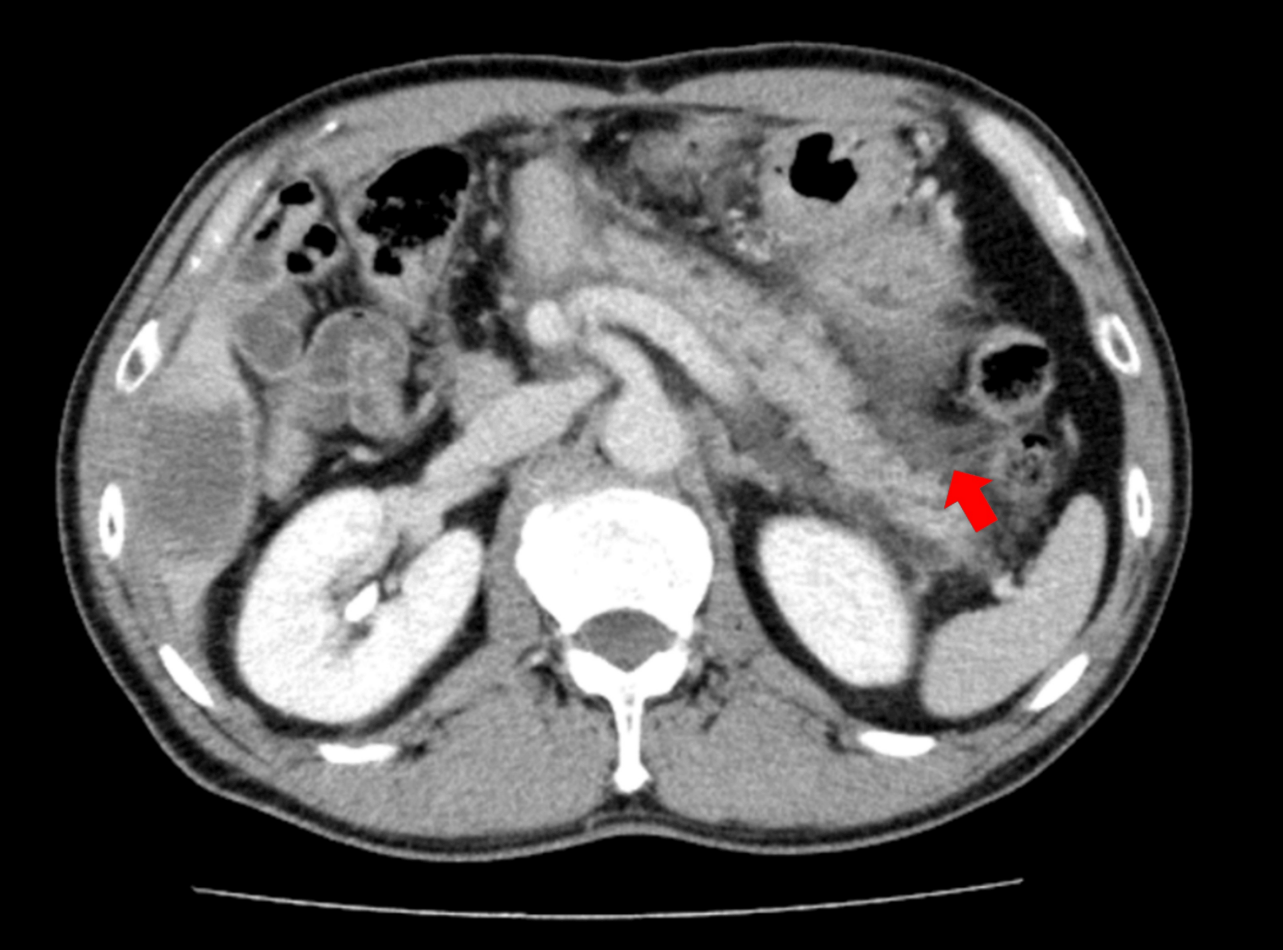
Contrast‑enhanced computed tomography at the first episode of acute pancreatitis (March 2024) Contrast‑enhanced computed tomography obtained in March 2024 during the first episode of acute pancreatitis shows inflammatory changes and peripancreatic fat stranding consistent with acute pancreatitis.
